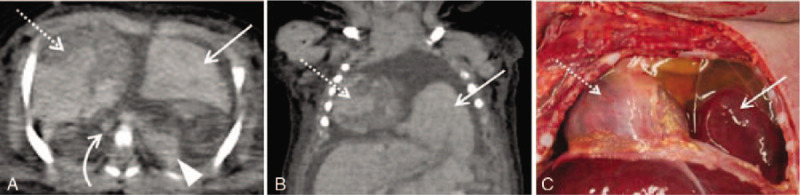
Representative case involving a deceased boy aged 1 day. Computed tomography (CT) was performed 17 h and 25 min after death. (A) Postmortem CT (PMCT) shows that the left thoracic cavity contains multiple organs such as the lateral segment of the left hepatic lobe (arrow), spleen (arrowhead), and a part of the intestine. The heart (dotted arrow) and thoracic aorta (curved arrow) are shifted to the right. (B) A coronal multiplanar reconstruction image proves useful for determining the orientation of the organs (arrow, lateral segment of the left hepatic lobe; dotted arrow, heart). Left congenital diaphragmatic hernia was suspected as a major pathological abnormality. Respiratory failure was considered the immediate cause of death. (C) Autopsy reveals left congenital diaphragmatic hernia and herniated structures, including the lateral segment of the left hepatic lobe (arrow) and heart (dotted arrow). Respiratory failure was confirmed as the immediate cause of death.
